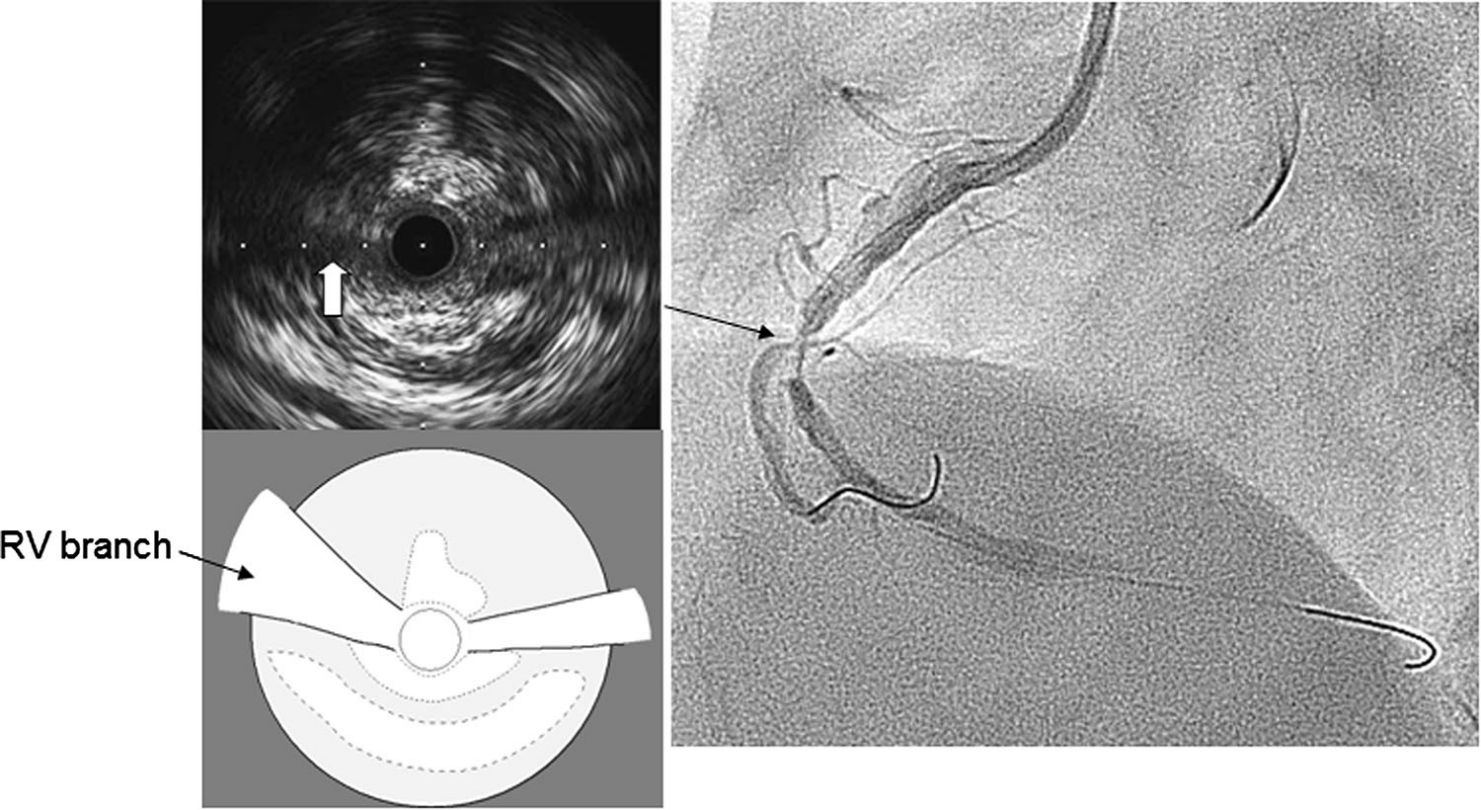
Angiogram and intravascular ultrasound (IVUS) image (Opticross; Boston scientific, Japan) at the right ventricular (RV) branch bifurcation after guidewire crossing and suction. IVUS image revealed that culprit lesion included attenuated plaque, and the RV branch was running through the plaque (*white arrow*). A small branch was also seen at the opposite side of RV branch by the angiogram and IVUS
